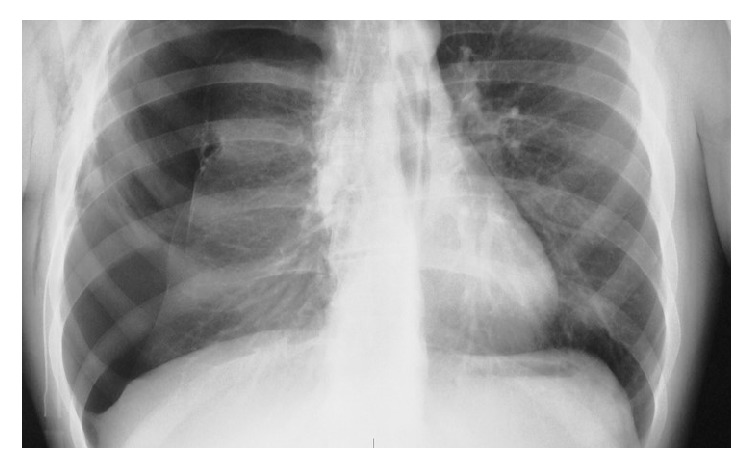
Operating theater chest X-ray with right pneumothorax.
